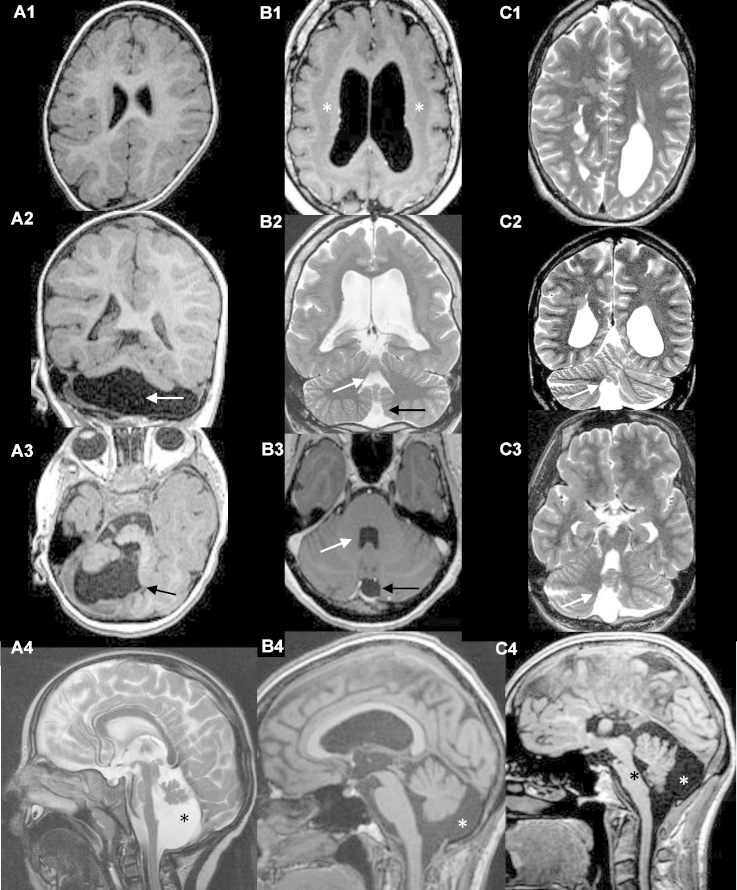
Cerebellar hypoplasia. First column (A1–4) – severe cerebellar hypoplasia. (A1) Axial T1-weighted image shows microcephaly, (A2) coronal T1 – weighted image shows enlarged posterior fossa (white arrow) with severely hypoplastic vermis and cerebellar hemispheres, (A3) T1-weighted axial image with large posterior fossa cerebro-spinal fluid (CSF) collection (black arrow), (A4) midline sagittal T2-weighted image shows enlarged IV ventricle, large collection of CSF in posterior fossa (asterisk) and hypoplastic vermis. Second column (B1–4) – isolated hypoplasia of cerebellar vermis. (B1) Asterisks show subcortical band heterotopia on T1-weighted axial image; coronal T2-weighted (B2) and axial T1-weighted (B3) images show enlarged IV ventricle (white arrow) and large collection of CSF in posterior fossa (black arrow), (B4) T1-weighted sagittal image with hypoplastic vermis and large collection of CSF in posterior fossa (white asterisk). Third column (C1–4) – severe hypoplasia of brainstem and cerebellum. (C1) Axial T2-weighted image, periventricular nodular heterotopia on the right; coronal (C2) and axial (C3) T2-weighted images show hypoplastic cerebellar vermis with enlarged IV ventricle and large collection of CSF in posterior fossa (white arrow), (C4) atrophic pons (black asterisk), hypoplastic vermis with enlarged collection of CSF in posterior fossa (white asterisk) and agenesis of corpus callosum on sagittal T1-weighted image.
